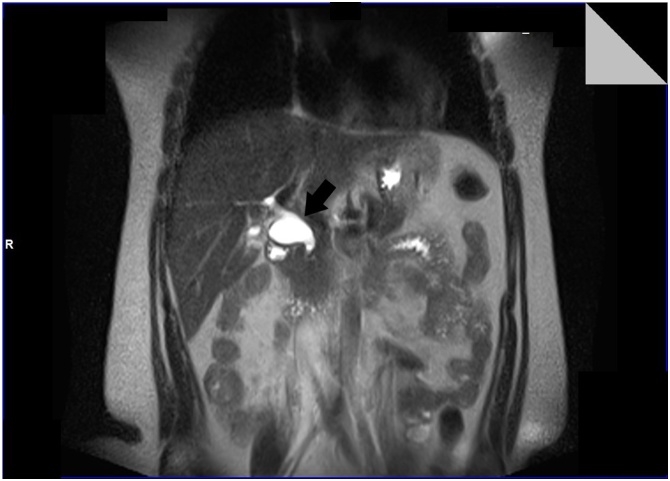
MRI showing fusiform dilatation of the proximal portion of the hepatocholococcus (arrow), measuring up to 2.5 cm, compatible with Todani type I choledochal cyst.
